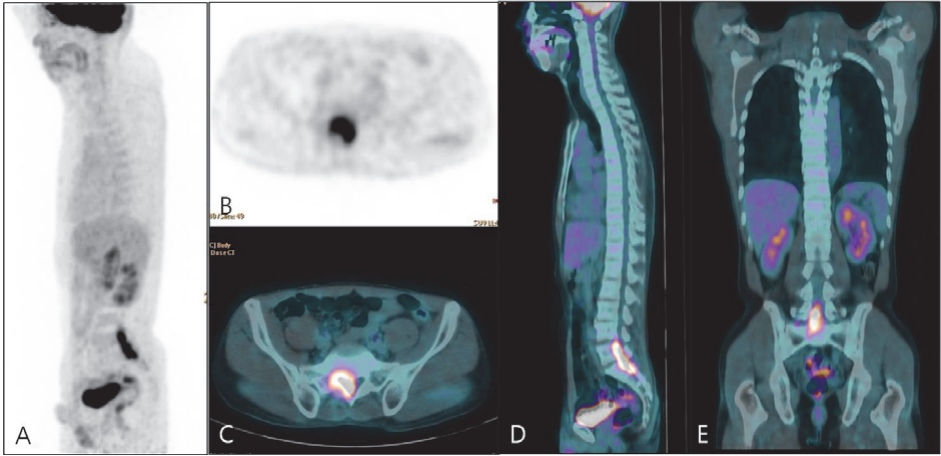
^18^F-FDG sagittal maximum intensity projection (MIP, A), axial PET (B), axial PET/CT (C), sagittal PET/CT (D), andcoronal PET/CT (E) images demonstrated intense FDG uptake (SUV_max_ 6.7), corresponding to the right spinal canal of the lumbosacral spine; there was no abnormal FDG uptake in other regions
